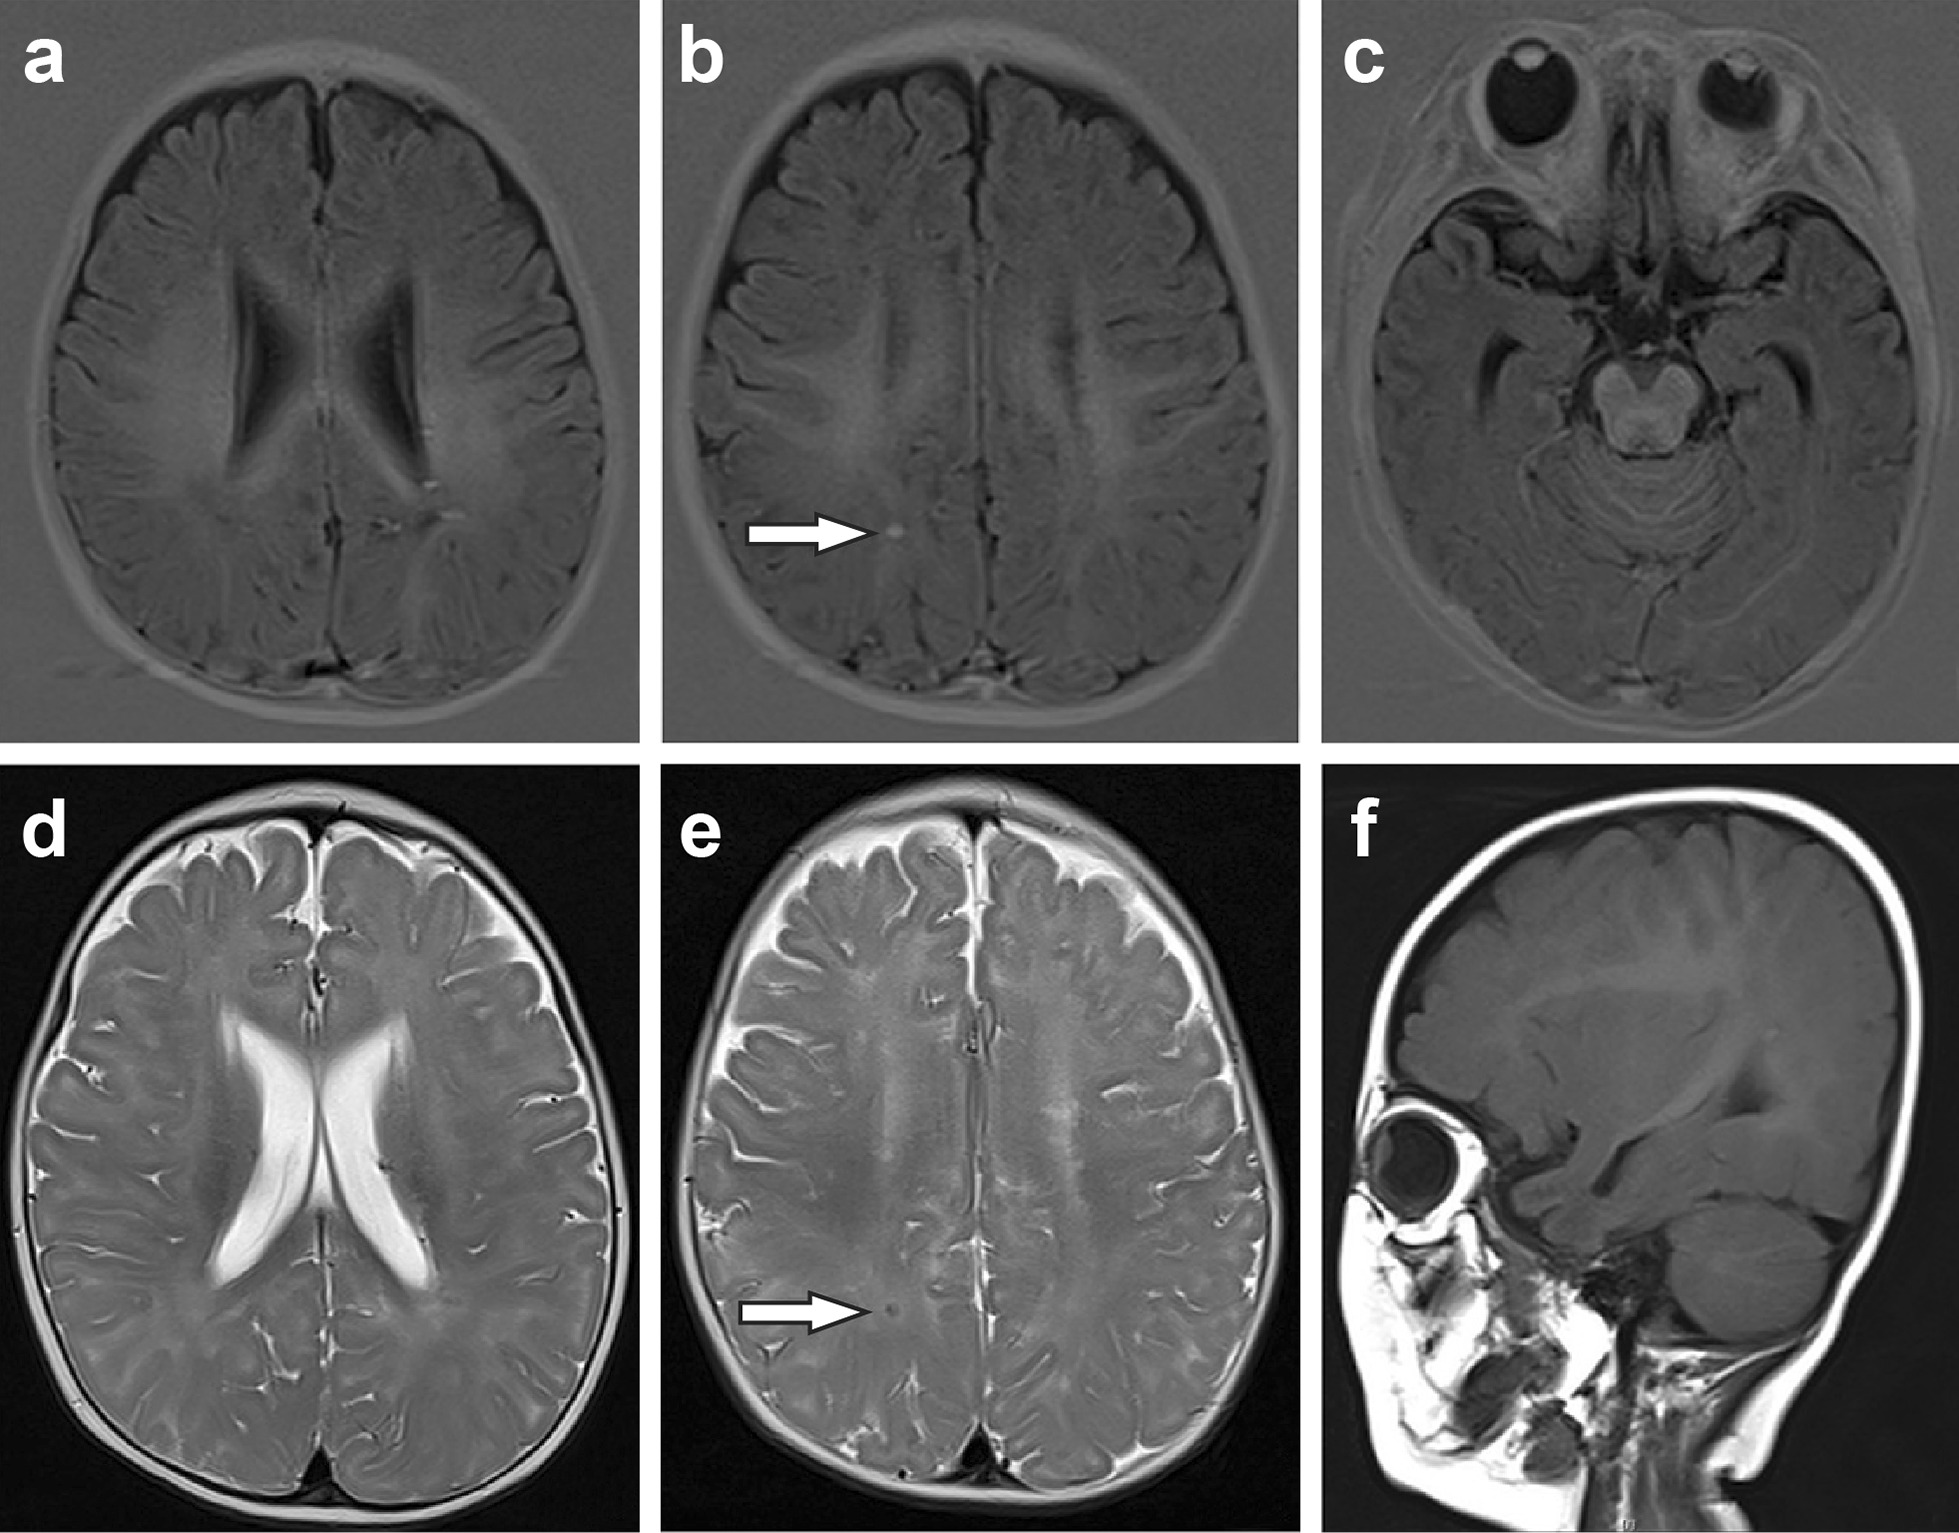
Second cranial MRI of the infant. A circular aberrant signal was observed in the white matter region of the posterior horn of the right lateral ventricle, with hyperintensity on T1-weighted images (T1WIs) (white arrows in panel b) and hypointensity on T2WIs (white arrows in panel e). There was no dilation of the ventricular system, no widening of sulci and fissures, and the midline structures were in the middle. Panels a–c refer to T1WIs, panels d, e refer to T2WIs, and panel f refers to T1WIs
